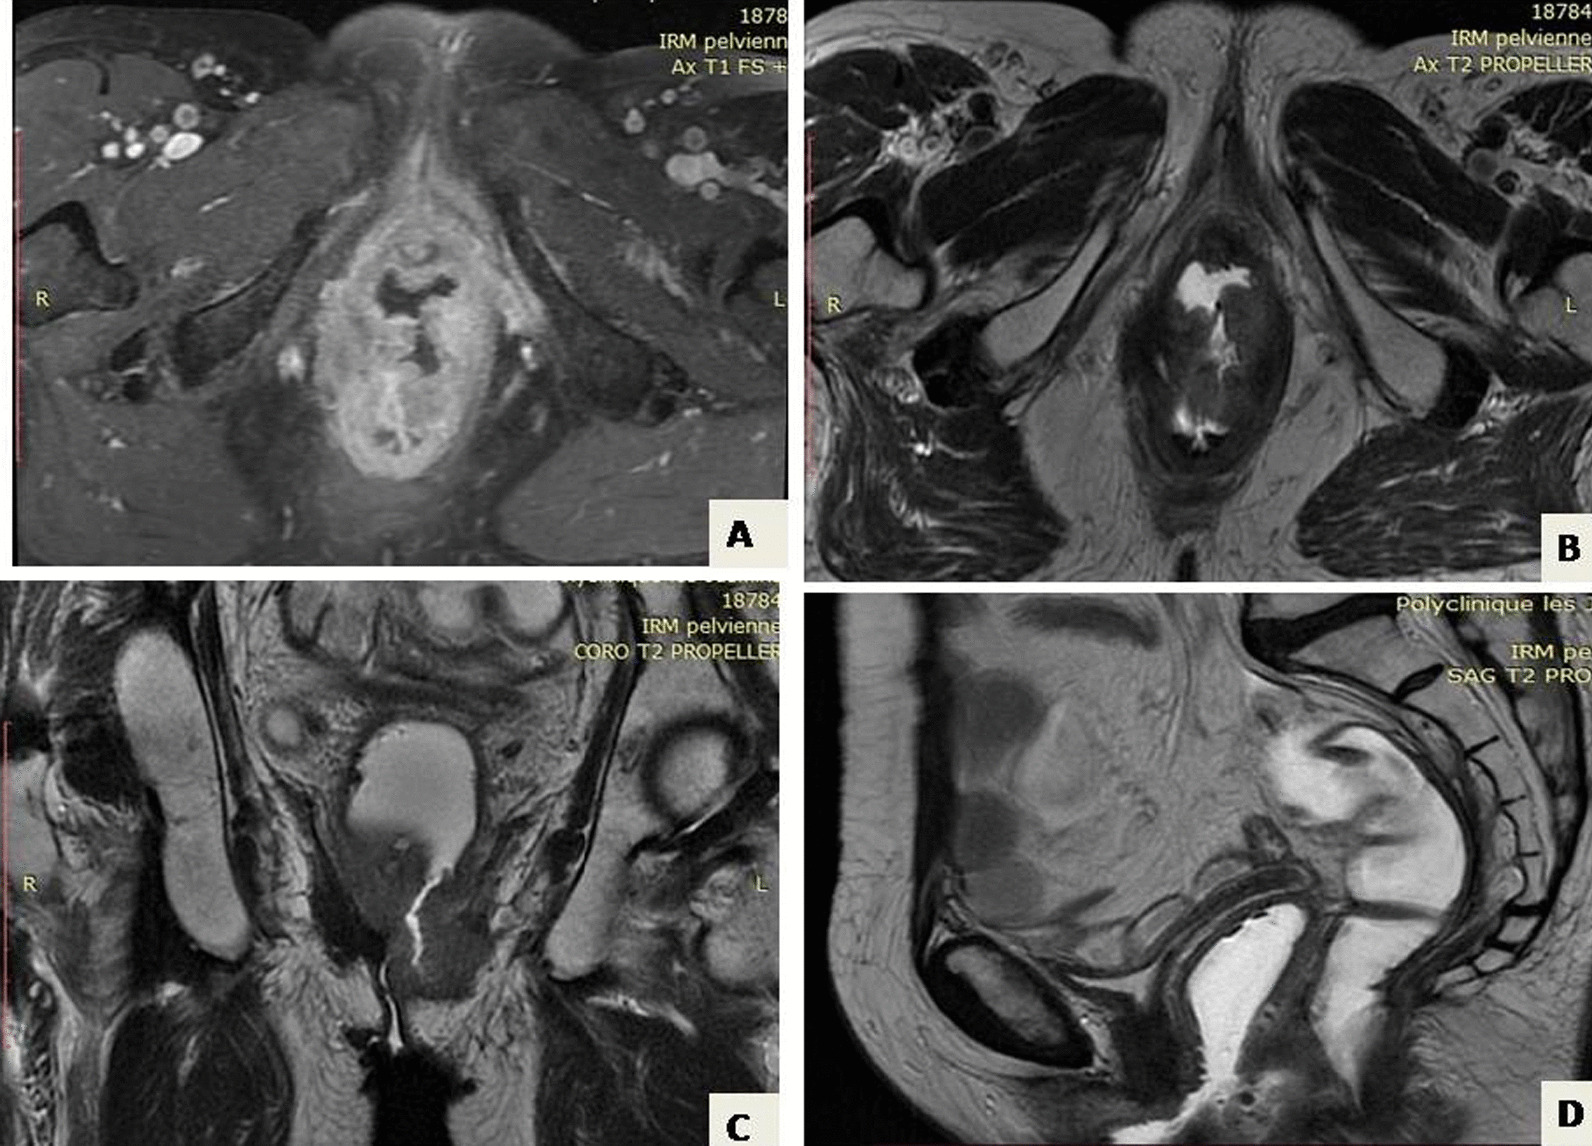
Pelvic MRI shows the vaginal tumor located in the posterior left wall of the vagina invading extrinsically into the rectum. A T1-weighted axial image shows the vaginal tumor. B T2-weighted axial image. C T2-weighted coronal image. D Sagittal view
